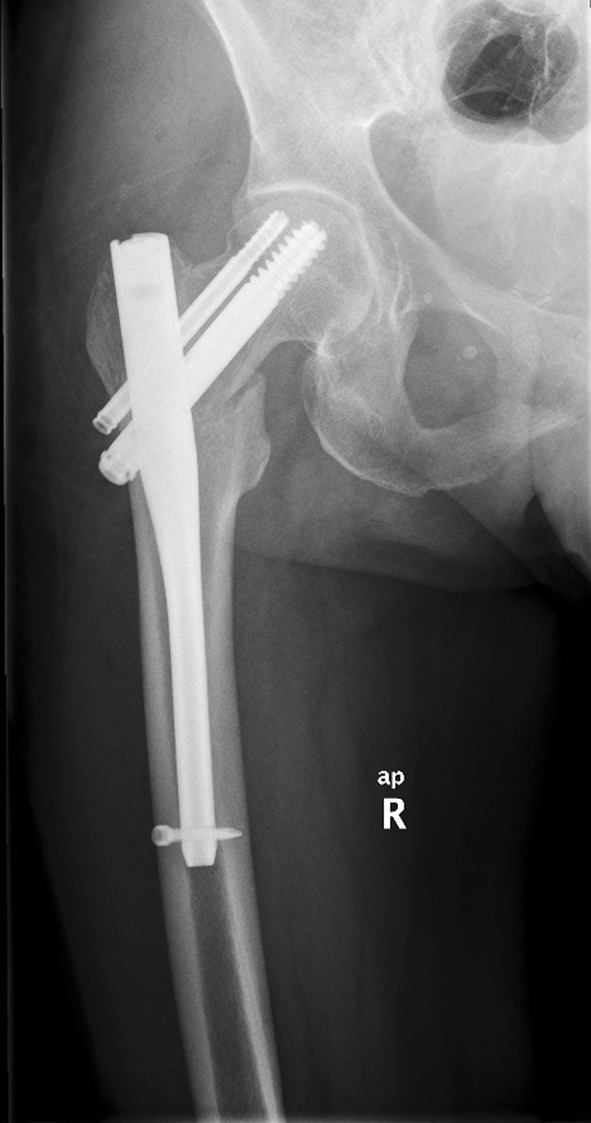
The anteroposterior radiograph after the surgery
